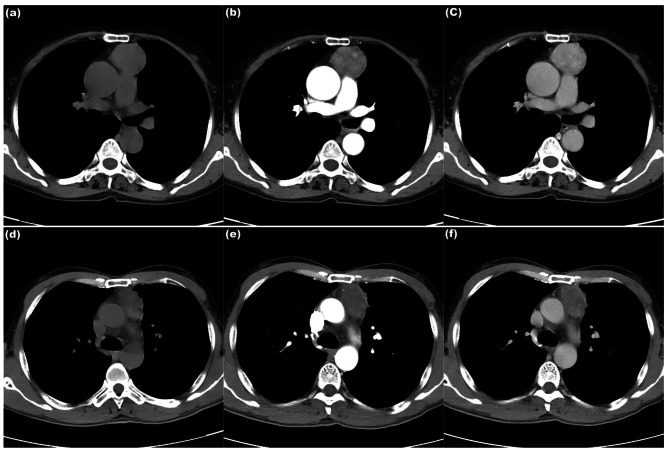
CT features of TETs on axial CT images of mediastinal window. (a)-(c) A 67-year-old female with type AB thymoma. Axial CT images show a round tumor with well-defined in anterior mediastinum. The CT values of the tumor on unenhanced scan (a), arterial phase (b) and venous phase (c) are 44.07 Hounsfeld Unit (HU), 95.15HU and 125.36HU, respectively, while the maximum enhanced CT value (CE_max_) of the tumor is 81.29HU. (d)-(f) A 50-year-old male with type B3 thymoma. Axial CT images show an oval tumor with ill-defined in anterior mediastinum. The CT values of the tumor on unenhanced scan (d), arterial phase (e) and venous phase (f) are 40.76HU, 45.62HU and 63.03HU, respectively, while the CE_max_ of the tumor is 22.27HU
